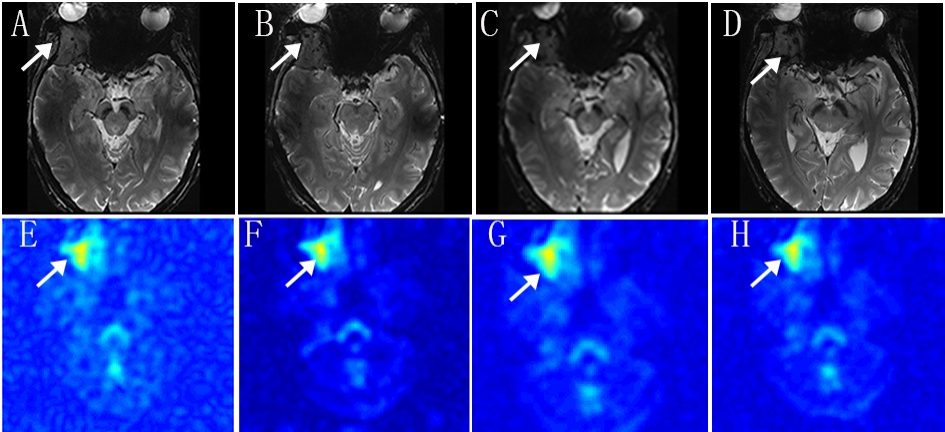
T2-weight imaging and sodium MRI. Axial T2-weight MRI with pretreatment (A), 48 hours after treatment (B), post-treatment of 1 week (C) and post-treatment of 1 month (D). Axial Sodium MRI Images with pretreatment (E)  48 hours after treatment (B), post-treatment of 1 week (C), post-treatment of 1 month (D)
